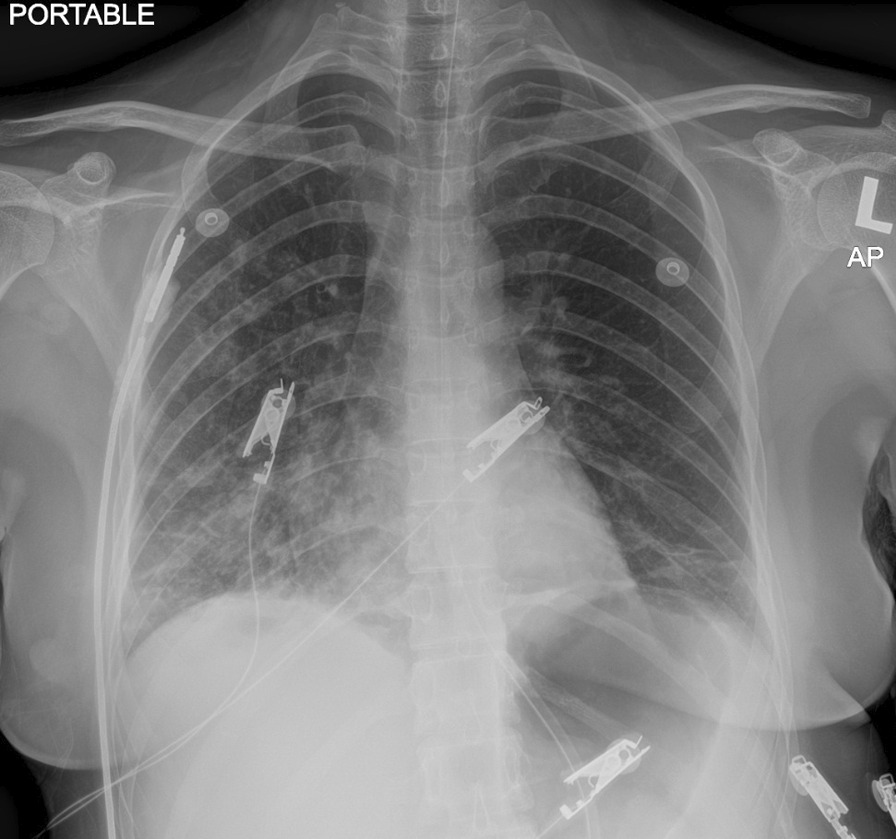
Radiographic imaging of chest taken on second day of admission when the patient developed shortness of breath, showing heterogeneous opacity over the lower right zone
